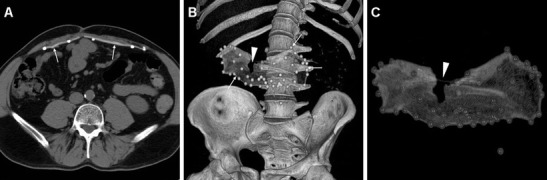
CT assessment of a 15x9 cm Gore-Tex Dual-Mesh® PM positioned laparoscopically 18 months earlier after previous failed attempts to repair an epigastric hernia. The ePTFE PM (thin arrows) secured by metallic-attenuation sutures is easily recognized on unenhanced axial CT image (a), and well depicted in its entirety and reticular structure by three-dimensional volume-rendering reconstructions (b, c). Additionally, a clinically unsuspected focal breach (arrowheads) was noted at its upper aspect, probably resulting from excessive tension
